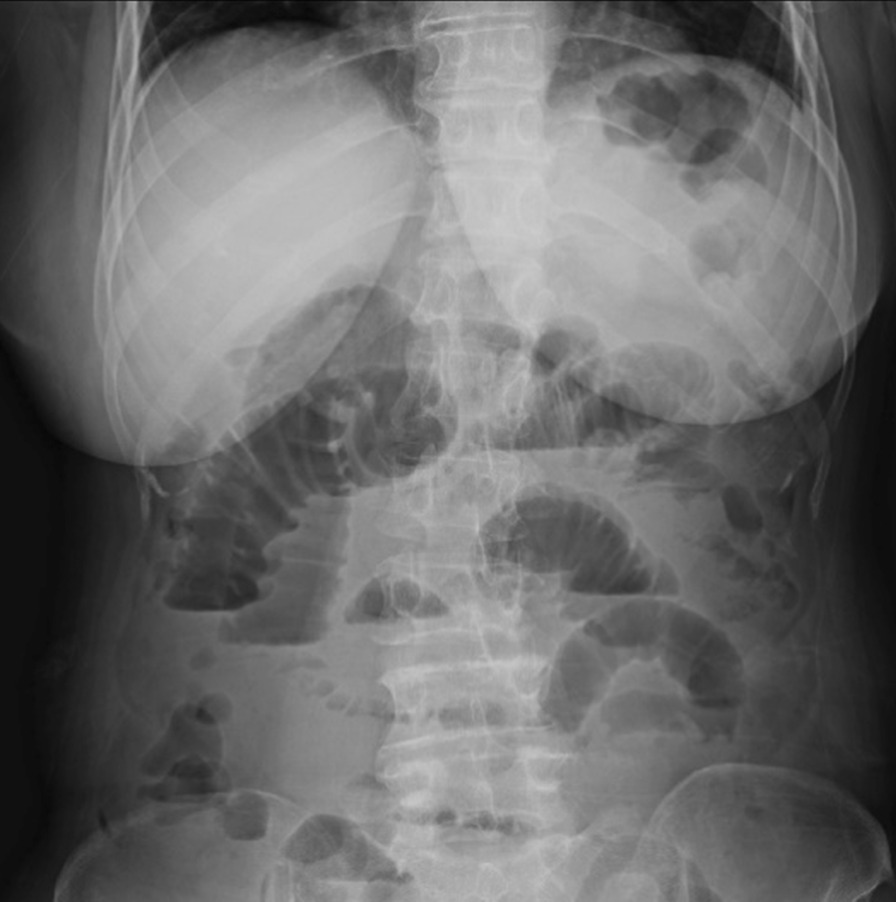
Abdominal X-ray demonstrating niveau formation in 6th POD
